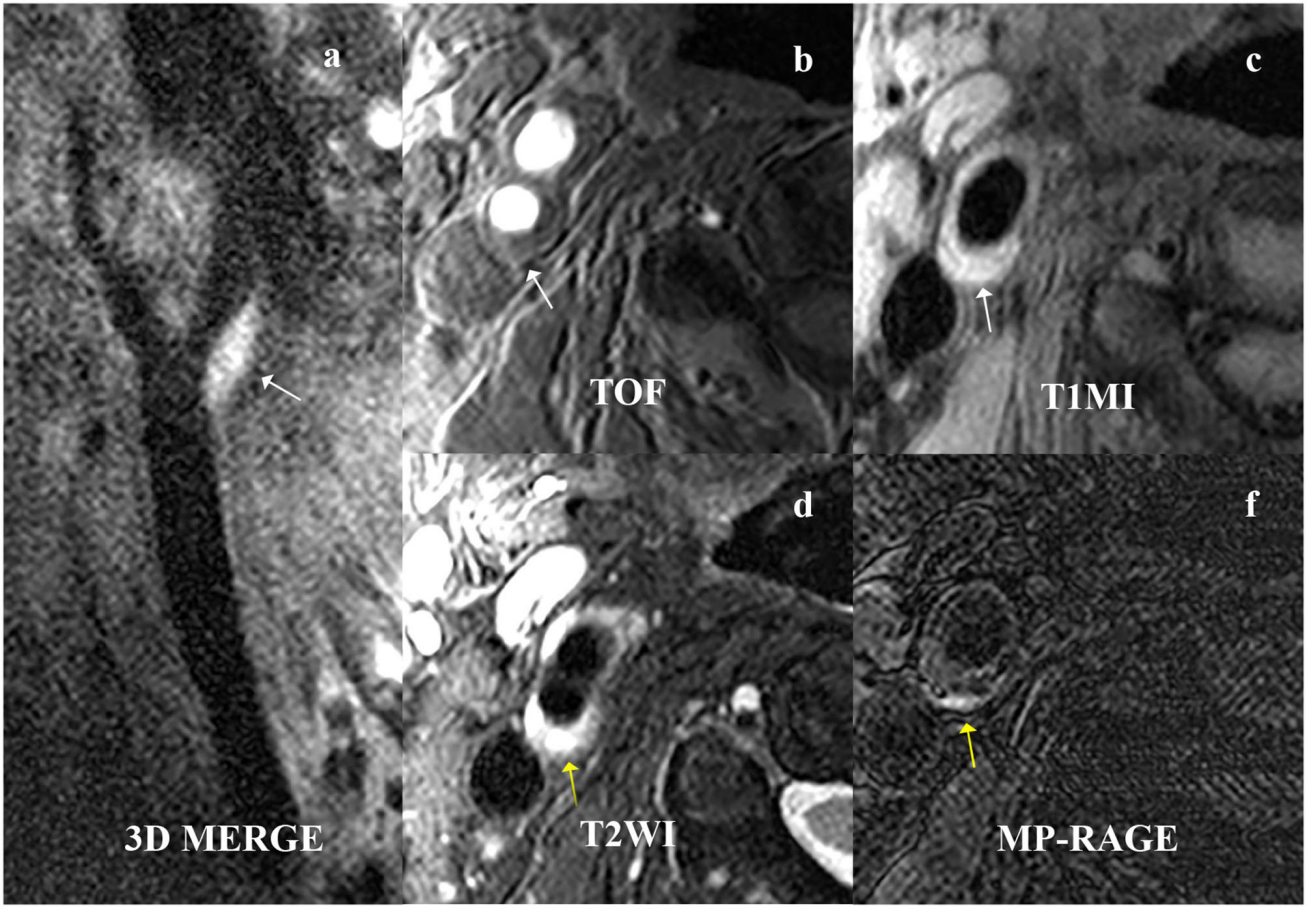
A 61-year-old male patient presented with right ischemic stroke. The plaque is shown at the beginning of the right internal carotid artery on (a) 3D MERGE, (b) TOF, and (c) T1W (white arrow). The intraplaque hemorrhage (IPH) is characterized by hyperintensities on (d) T2WI and (e) MPRAGE (yellow arrow). 3D MERG, 3D multiple-echo recalled gradient-echo; 3D-TOF, three-dimensional time of flight; T1W, T1-weighted; T2W, T2-weighted; MPRAGE, magnetization-prepared rapid acquisition gradient-echo.
